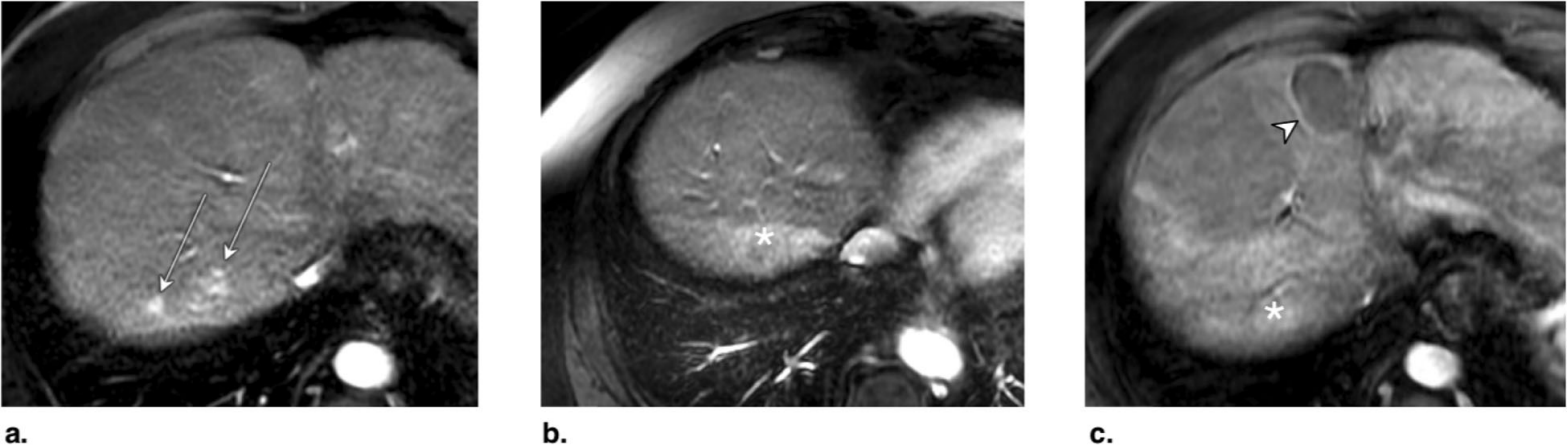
Axial T1-weighted MR in late arterial phase obtained (a) pretreatment: image shows two LR-4 observations (arrows) with nonrim arterial phase hyperenhancement (APHE). b 2 months post drug-eluting beads TACE (DEB-TACE): image shows regional APHE (asterisk). c 10 months post DEB-TACE: image shows decrease in regional APHE (asterisk). No nodular enhancement nor washout is visible. The treated observation is categorized LR-TR nonviable. Note the radiofrequency ablation cavity of another treated lesion (arrowhead)
